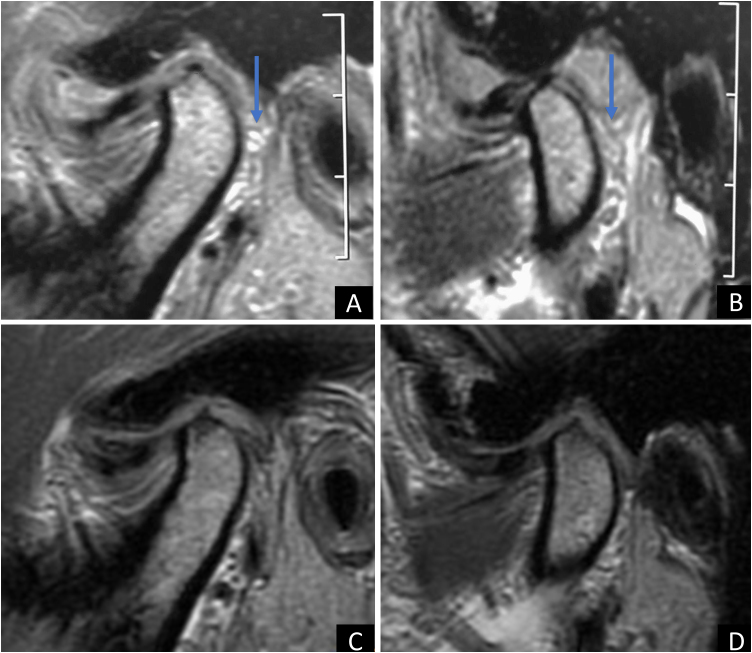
TMJ MRI. In A, mouth closed and B mouth open in the preoperative period. In C, mouth closed and D mouth open within 6-months of surgery.
